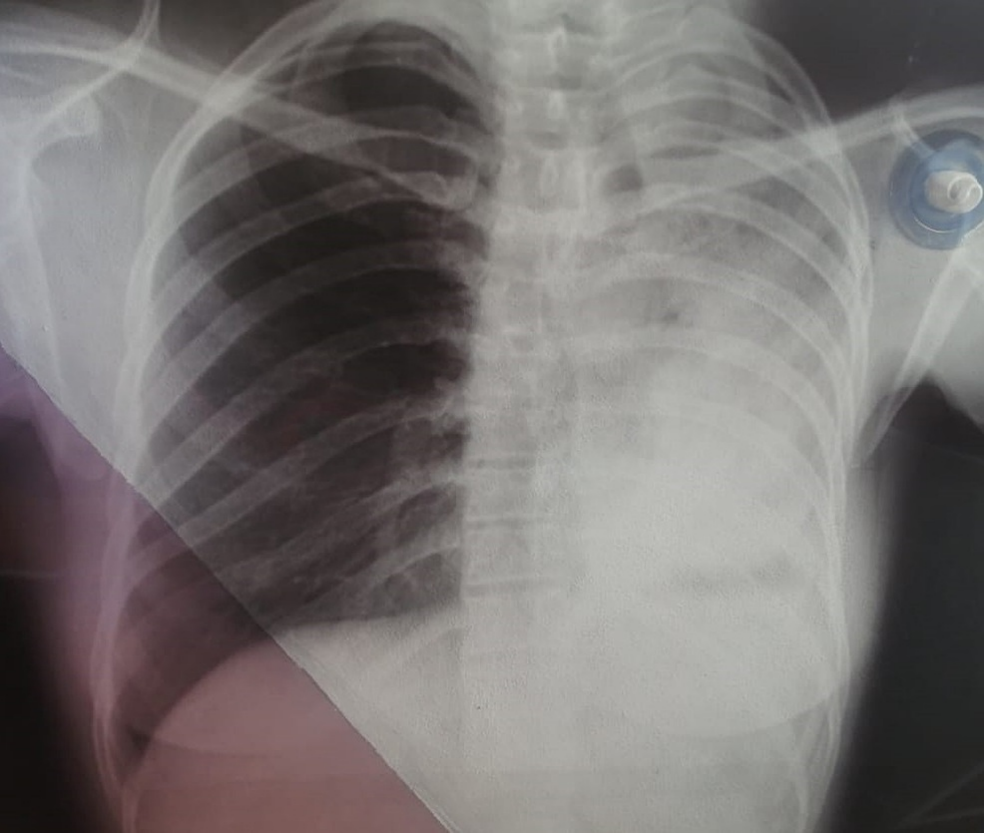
Chest X-ray showing complete fibrosis of the left lung.
